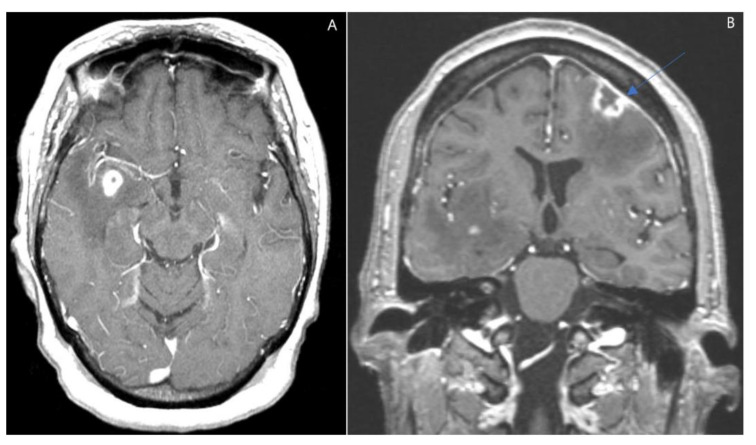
(A,B) Axial and Coronal enhanced T1 weighted MRI. There are peripherally enhancing lesions within the left frontal and right temporal lobes and pachymeningeal enhancement (blue arrow, B) overlying the left frontal lesion.
